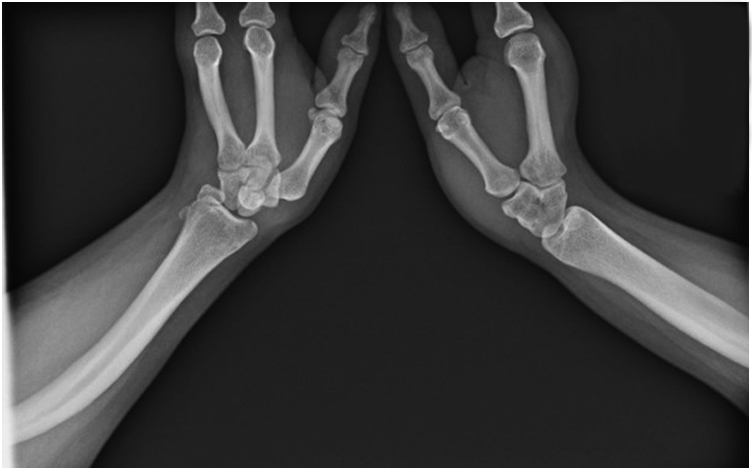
Bilateral wrist radiograph. A posteroanterior radiograph of the bilateral wrists demonstrates single bone forearms, presumed to be the radius, wrist coalitions, and the presence of rays 1–3 on the left side and rays 1–2 on the right side.
